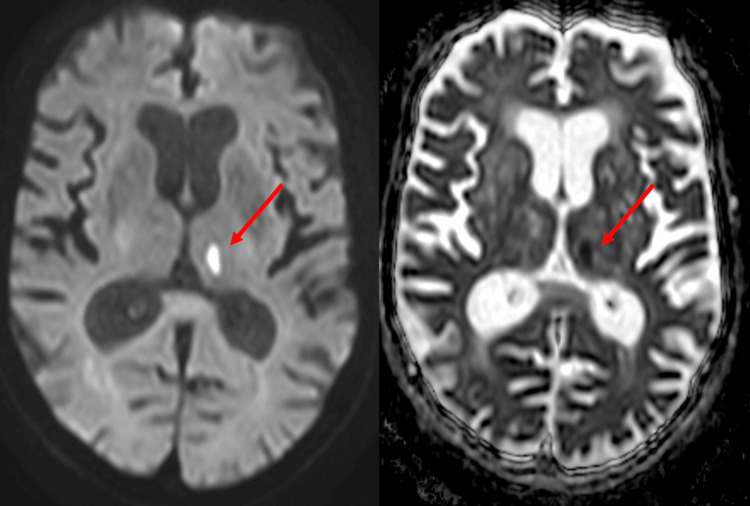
Magnetic resonance imaging (MRI) of the acute left thalamic infarct (indicated by red arrows), shown on diffusion-weighted imaging (DWI) (left), with an accompanying apparent diffusion coefficient (ADC) map (right)
